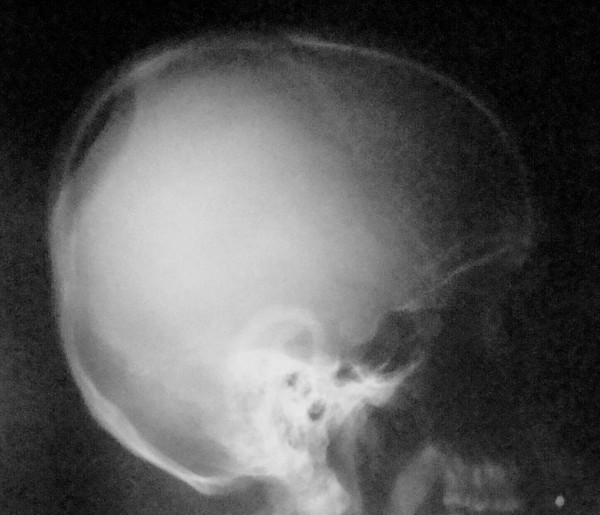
Plain radiograph of the skull depicting the osteolytic defect on the left temporal bone.
